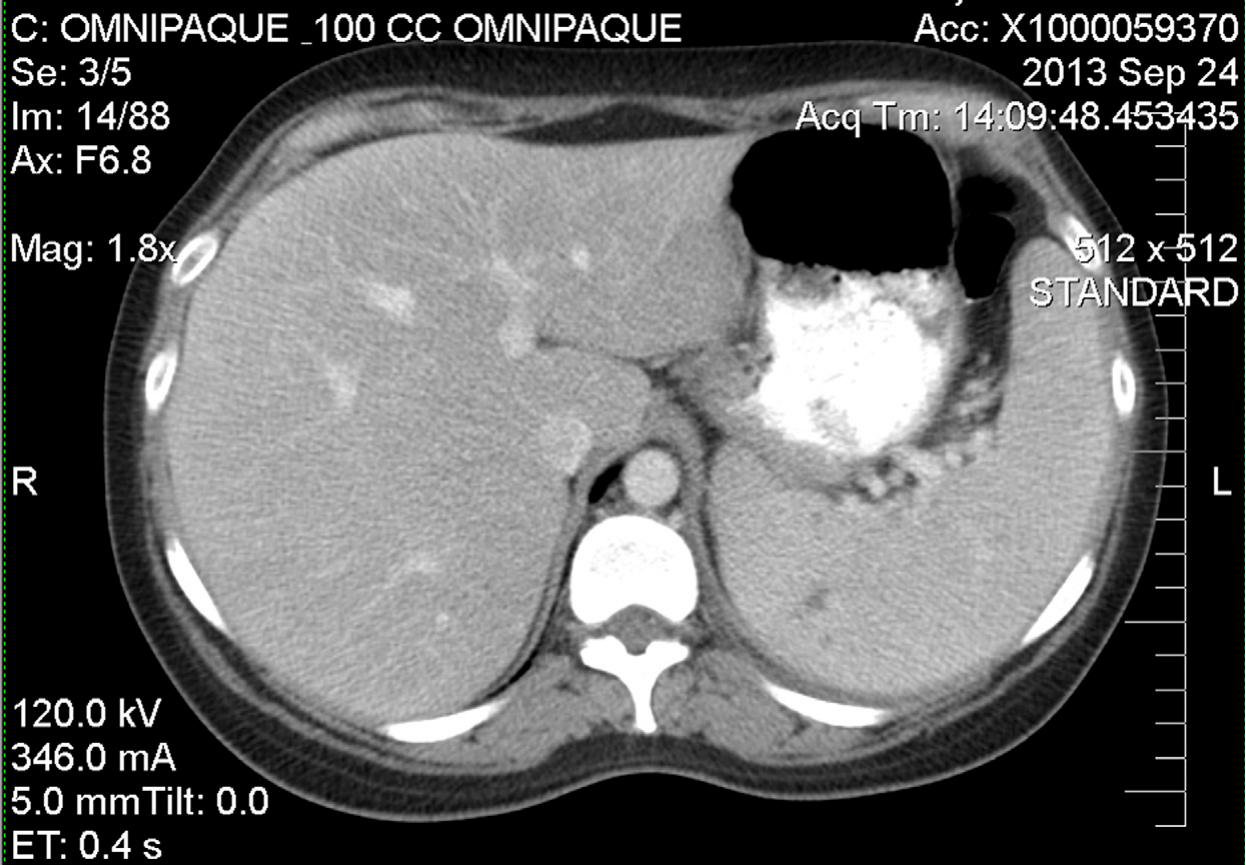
Abdominal CT showing splenomegaly.
